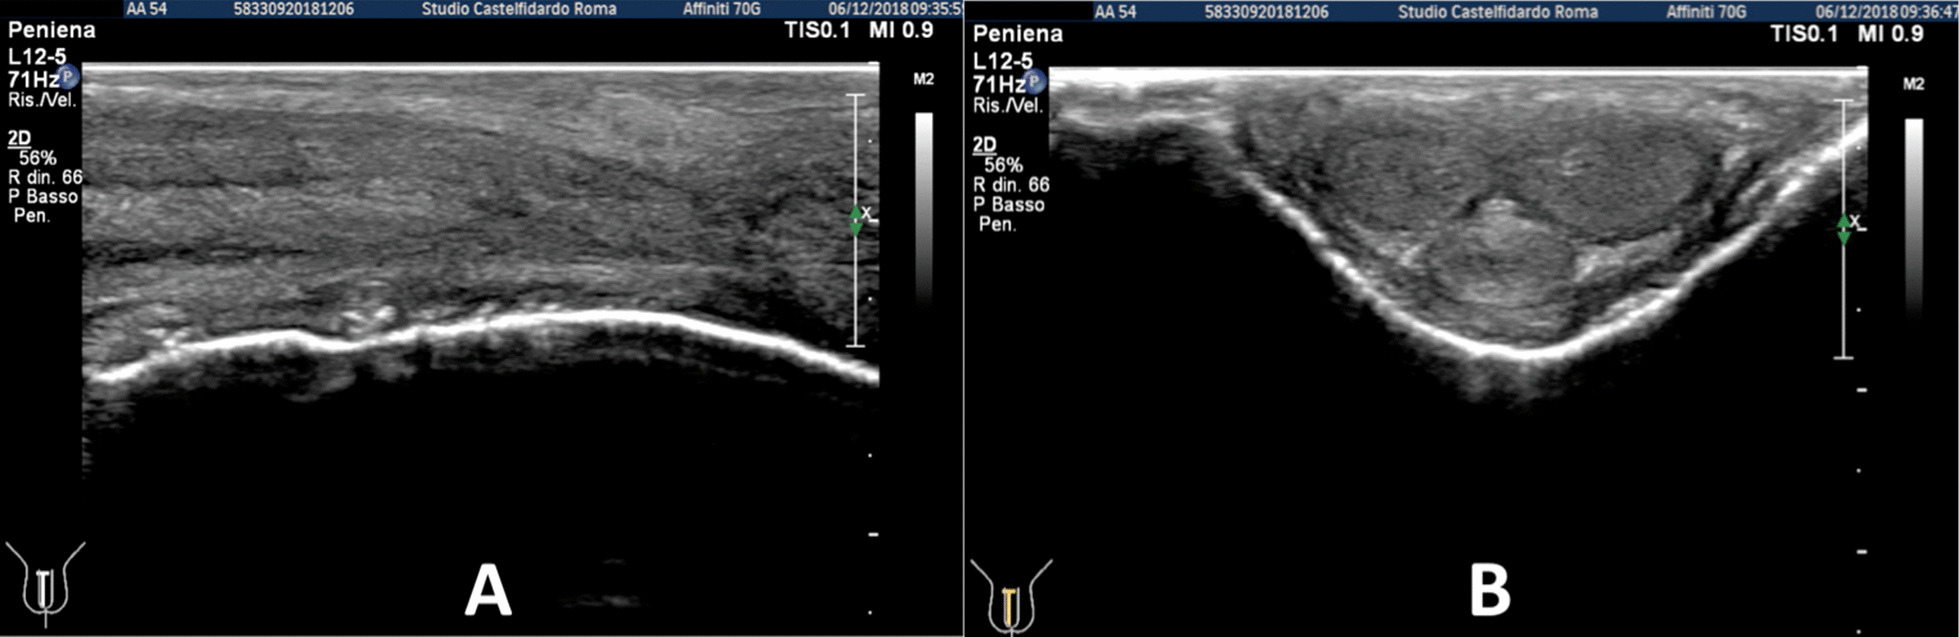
Penile ultrasound after the fifth treatment cycle (5 months). A Longitudinal scan. B Transverse scan
